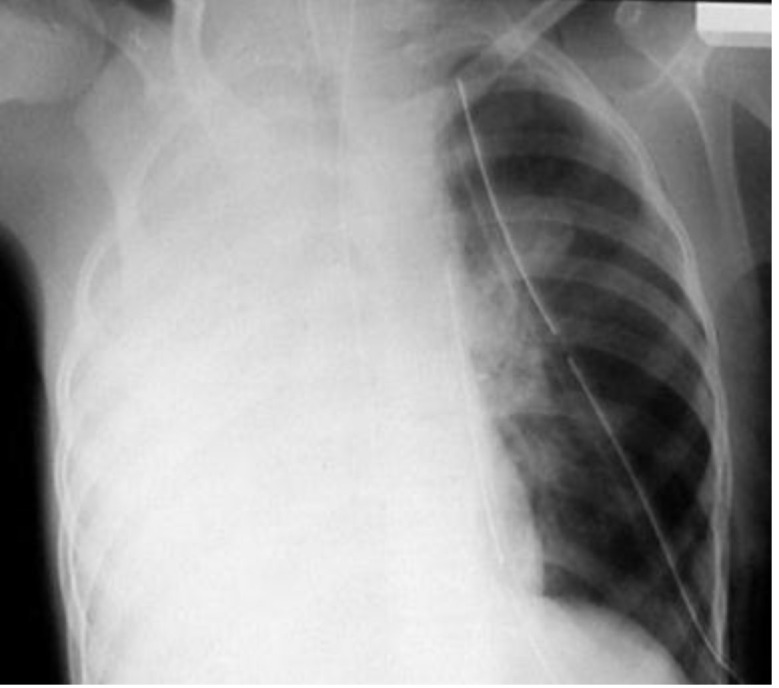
Postoperative chest X-ray: right lung ARDS
